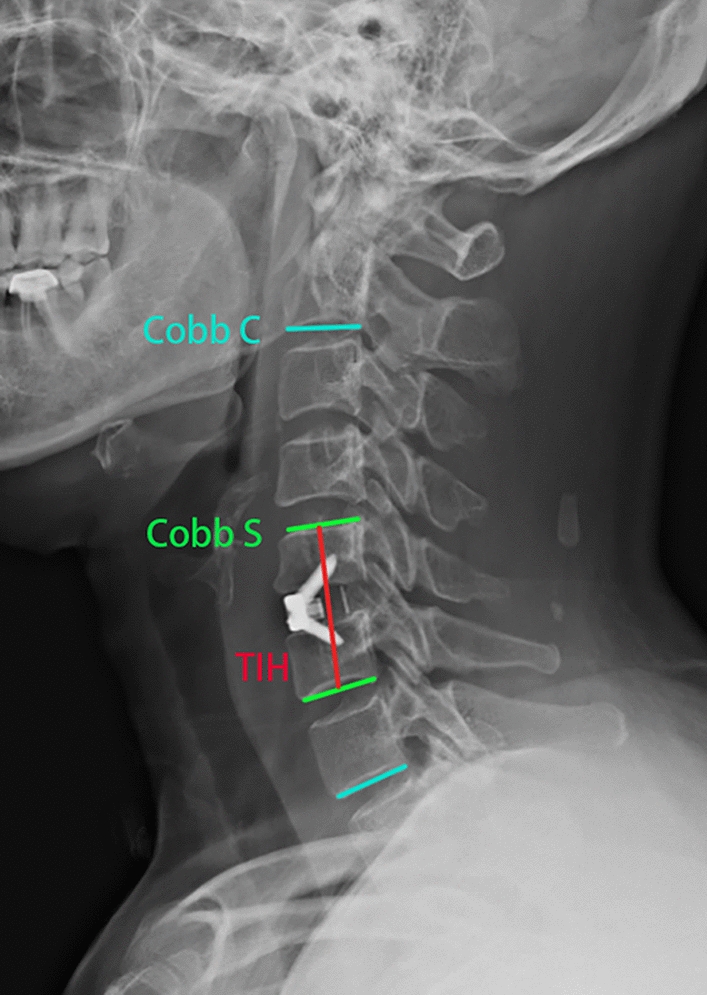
Lateral X-rays of the cervical vertebra. Cobb C: the Cobb angle between the lines perpendicular to the upper end plate of the C2 vertebral body and the lower end plate of the C7 vertebral body. Cobb S: the angle between a line perpendicular to the superior border of the upper affected vertebral body and the inferior border of the lower affected vertebral body. TIH: total interbody height
